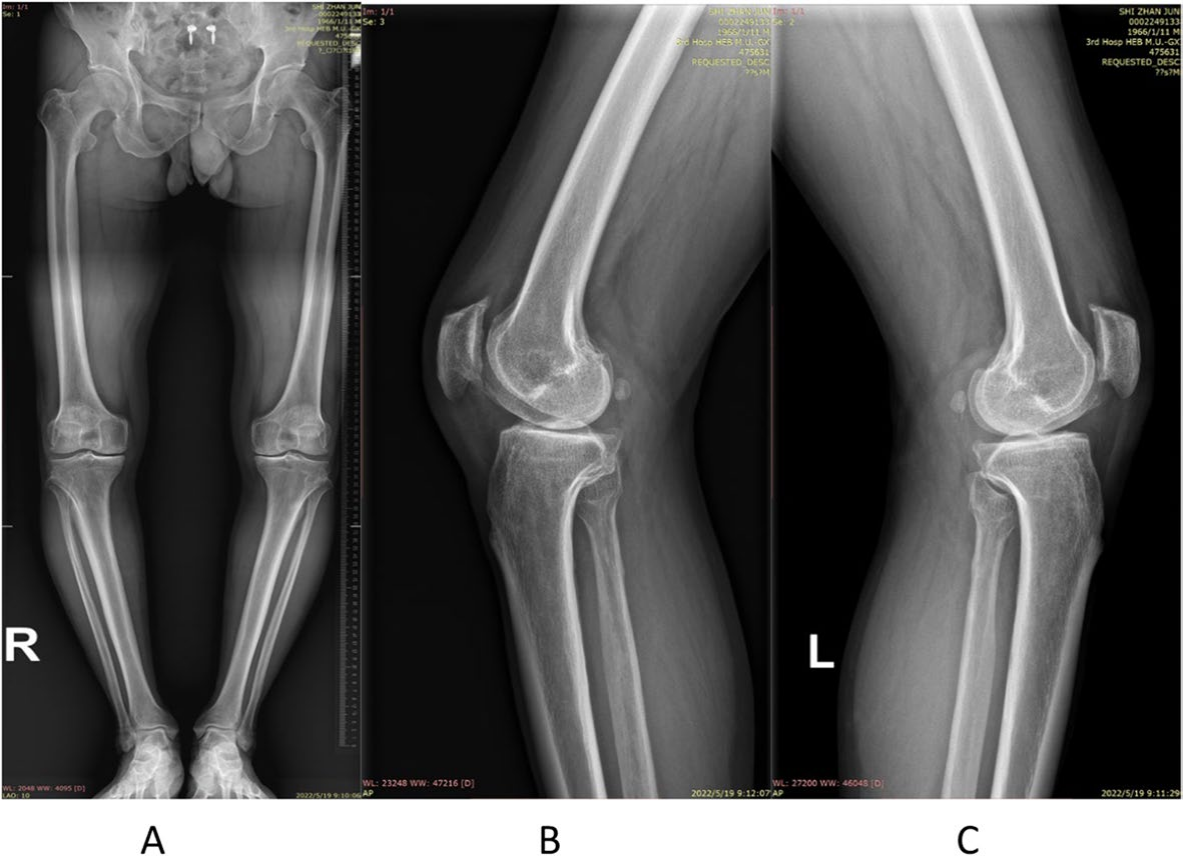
A-C Weight-bearing full-length radiographs of the lower limb and knee lateral x-ray before bilateral THO
